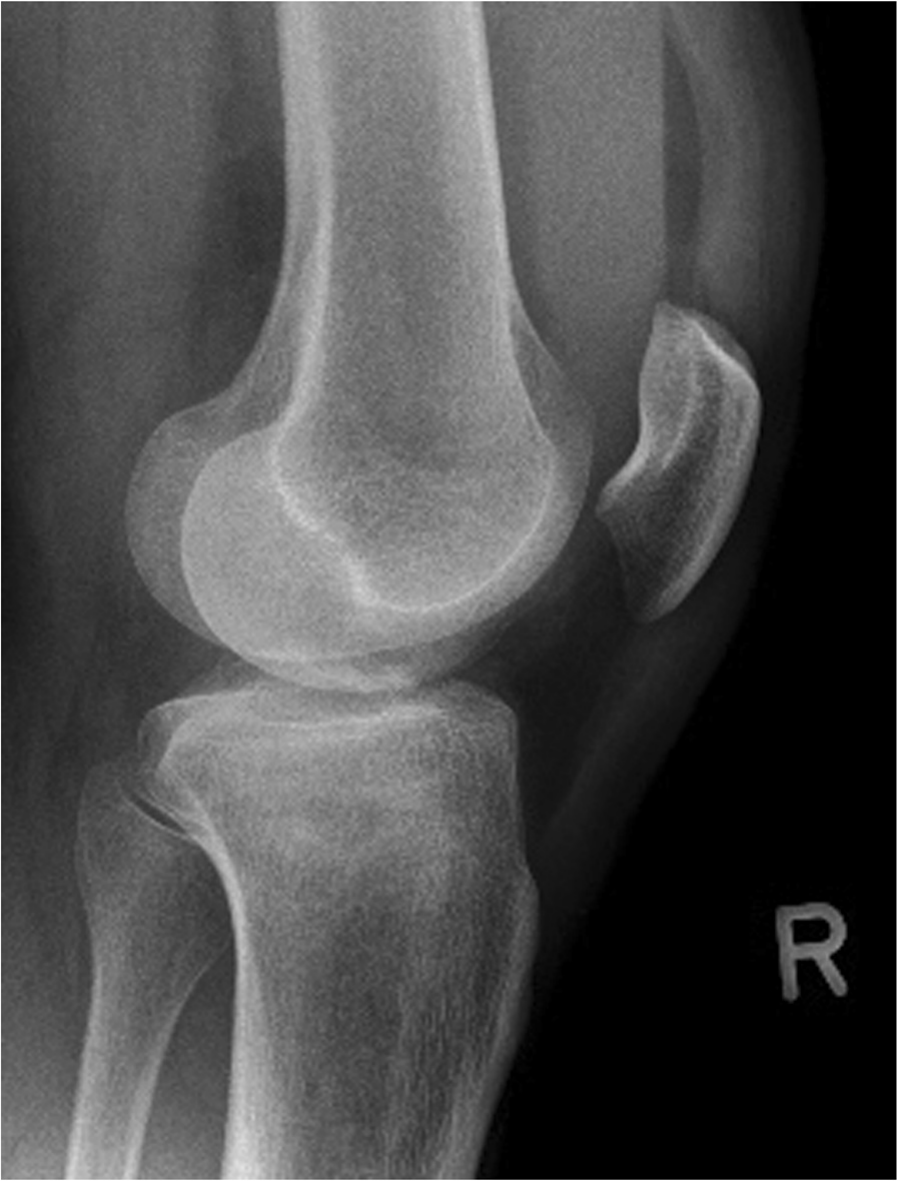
Lateral X-ray of the knee showing an AO 41 B3.1 fracture type.
